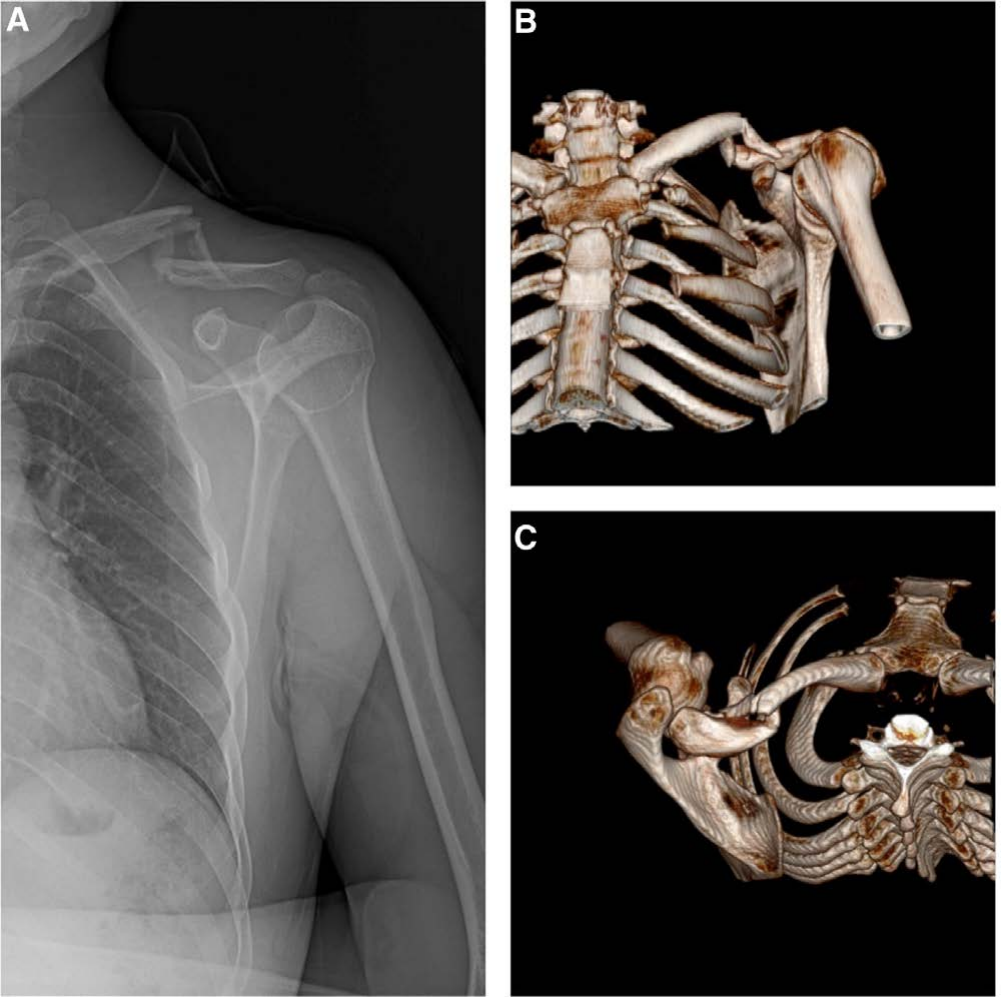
(A) Comminuted fracture of the clavicle, 30 years old, male patient, who fell from a height of about 2 meters, preoperative X-ray image. (B and C) 3-D computed tomography imaging for surgical planning.
